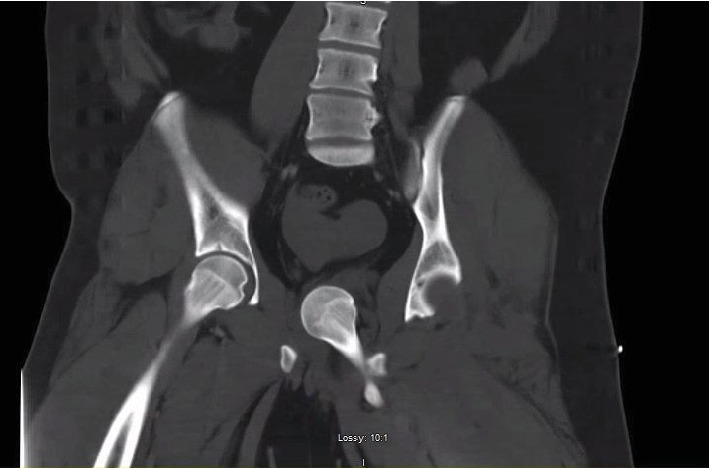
Coronal slice of CT abdomen and pelvis with contrast demonstrating medial displacement of femoral head into the true pelvis with empty acetabulum on the left.
